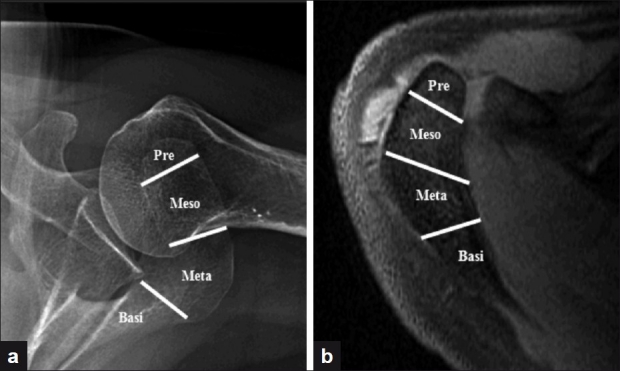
(a) Axillary radiograph; (b) Axial magnetic resonance image. (Pre – Preacromiale; Meso – Mesoacromiale; Meta – Metaacromiale; Basi – Basiacromiale)
